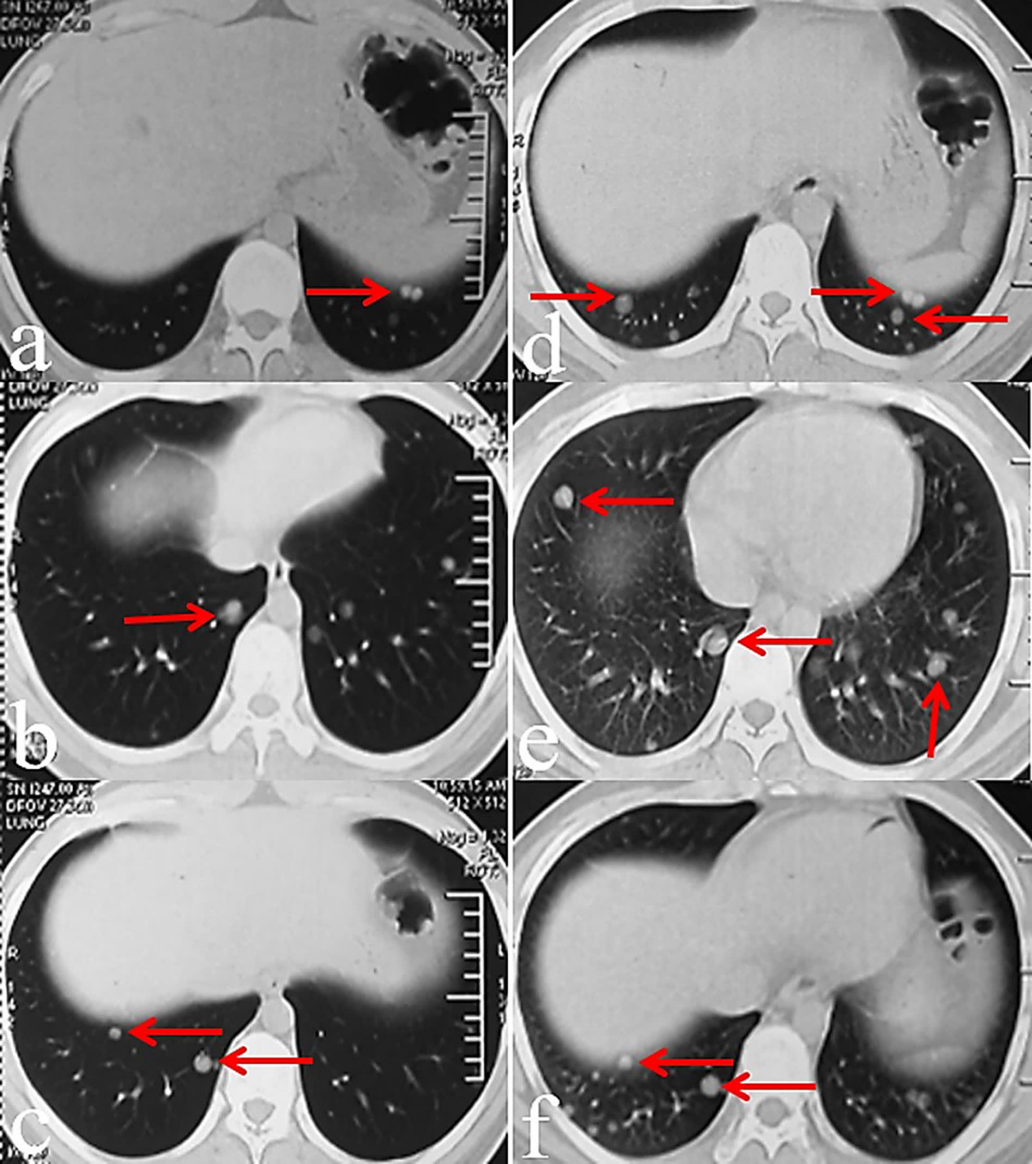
CT images before and after gemcitabine-docetaxel chemotherapy March 2015 CT images collected before gemcitabine-docetaxel chemotherapy, showing multi-lung metastatic nodules (a-c). May 2015 CT images collected after two gemcitabine-docetaxel chemotherapy cycles, showing disease progression presenting as increased lesion numbers and sizes (red arrows(d-f).
